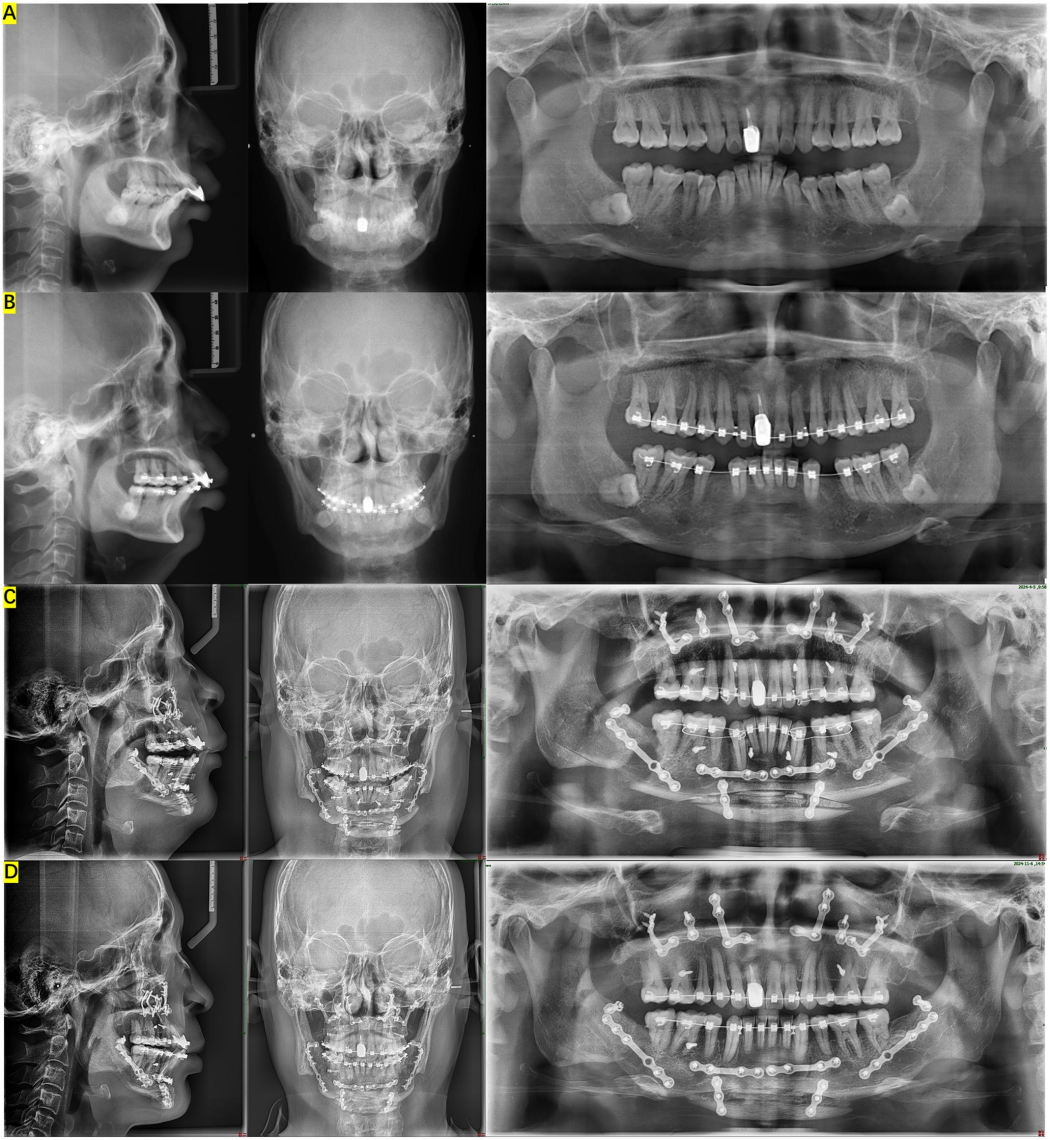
Lateral cephalometric radiograph, posteroanterior cephalometric radiograph and panoramic radiograph during orthodontic-surgical combined therapy. (A) Before pre-orthognathic orthodontics, (B) 1 week before orthognathic surgery, (C) 1 week after orthognathic surgery, (D) 6 months after orthognathic surgery.
